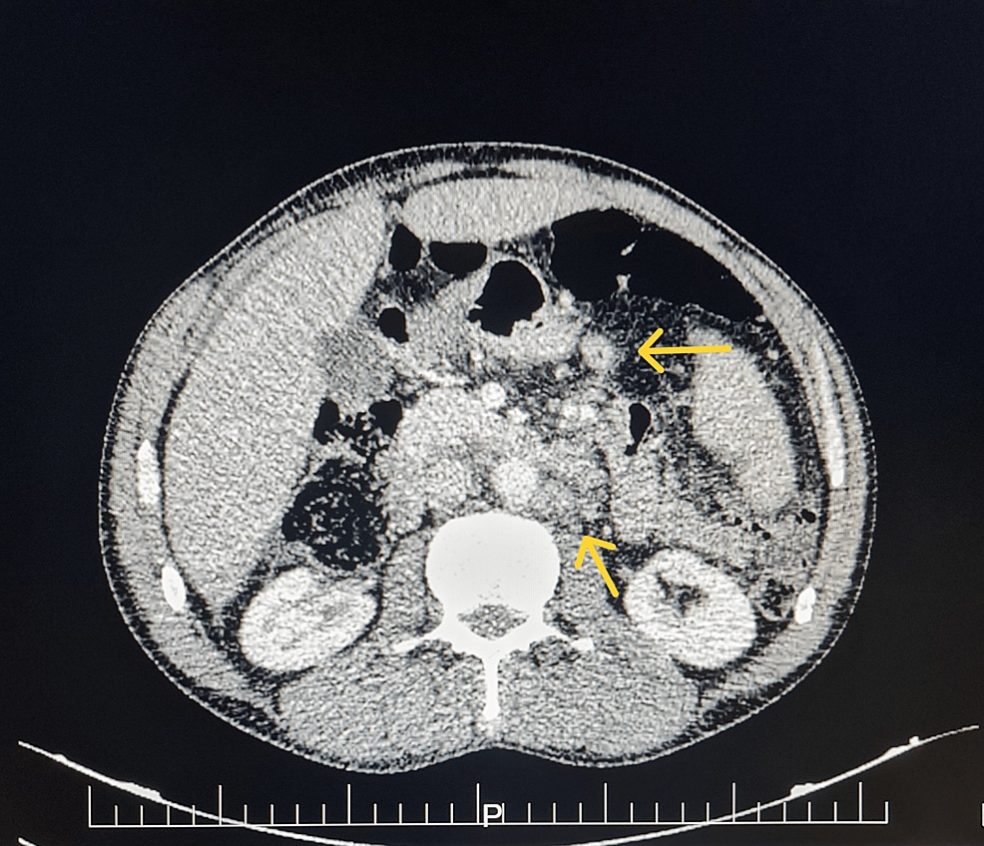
Axial CT images showing adenopathy below the diaphragm
